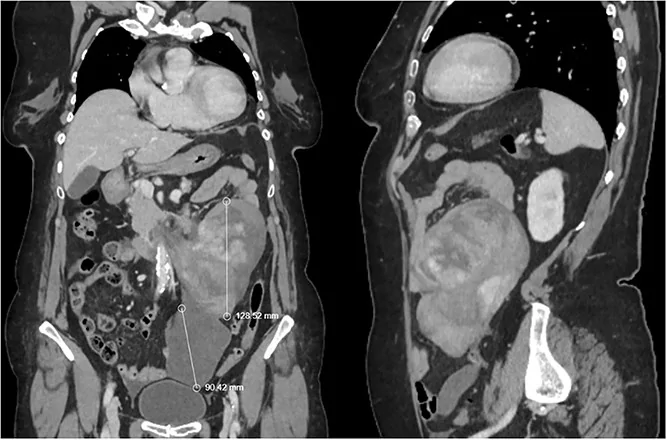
On the left, coronal CT-scan: at the top the retroperitoneal liposarcoma, below the mesenteric one, along with the corresponding maximum diameters; on the right, sagittal CT-scan: from top to bottom the retroperitoneal and mesenteric liposarcoma.
